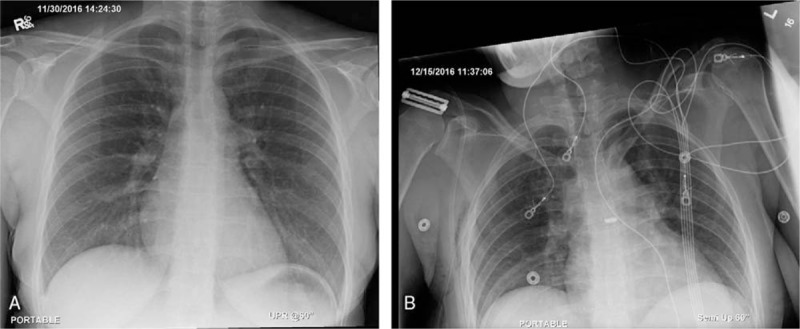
(A, B) Chest X-ray before and after surgery for case 1.
